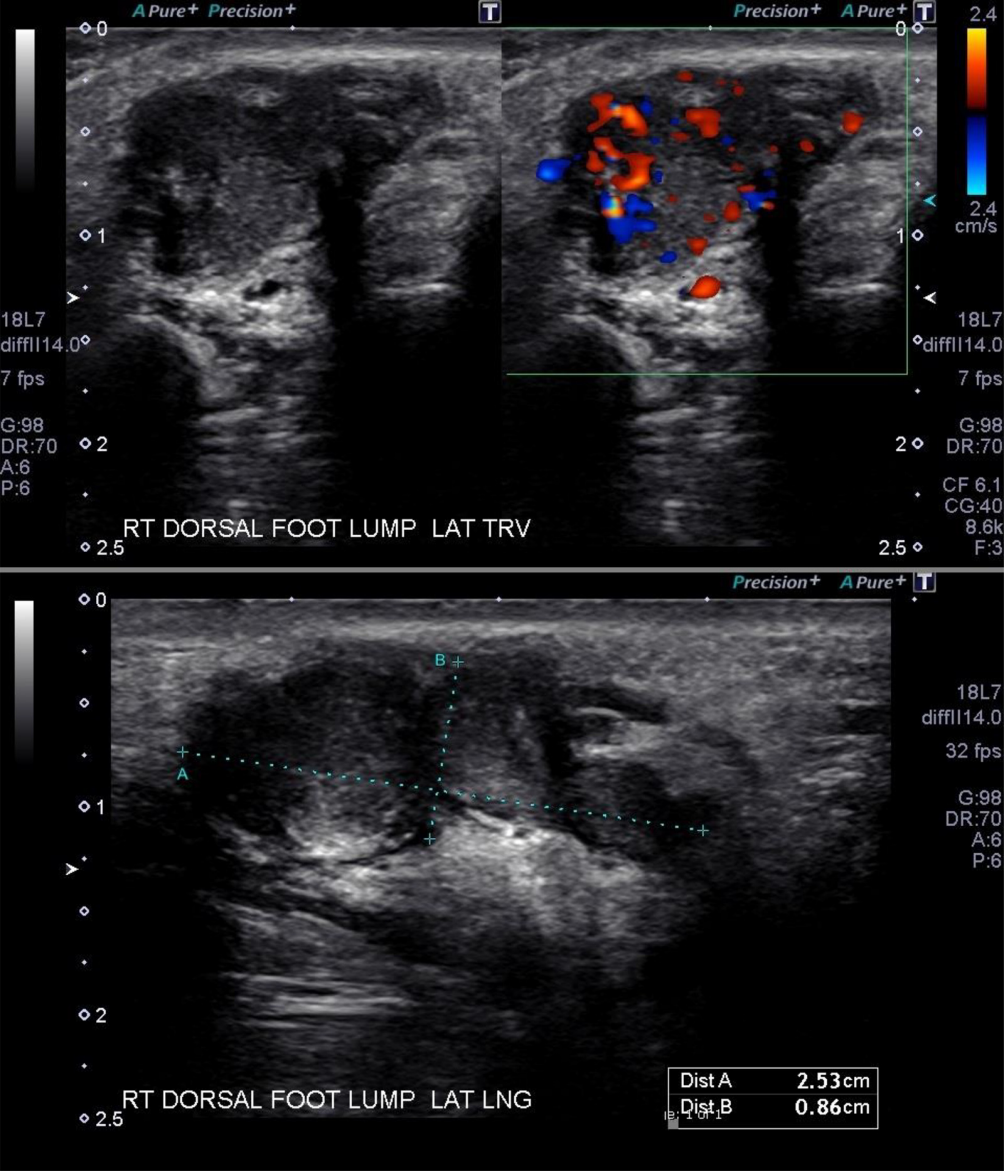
Lateral transverse without (upper left) and with (upper right) color Doppler ultrasound images demonstrate irregularly shaped cystic lesions with intralesional echogenic tissue with color Doppler. Longitudinal (lower) images demonstrate the longitudinal size of the lesion as seen above the level of the metatarsals.
